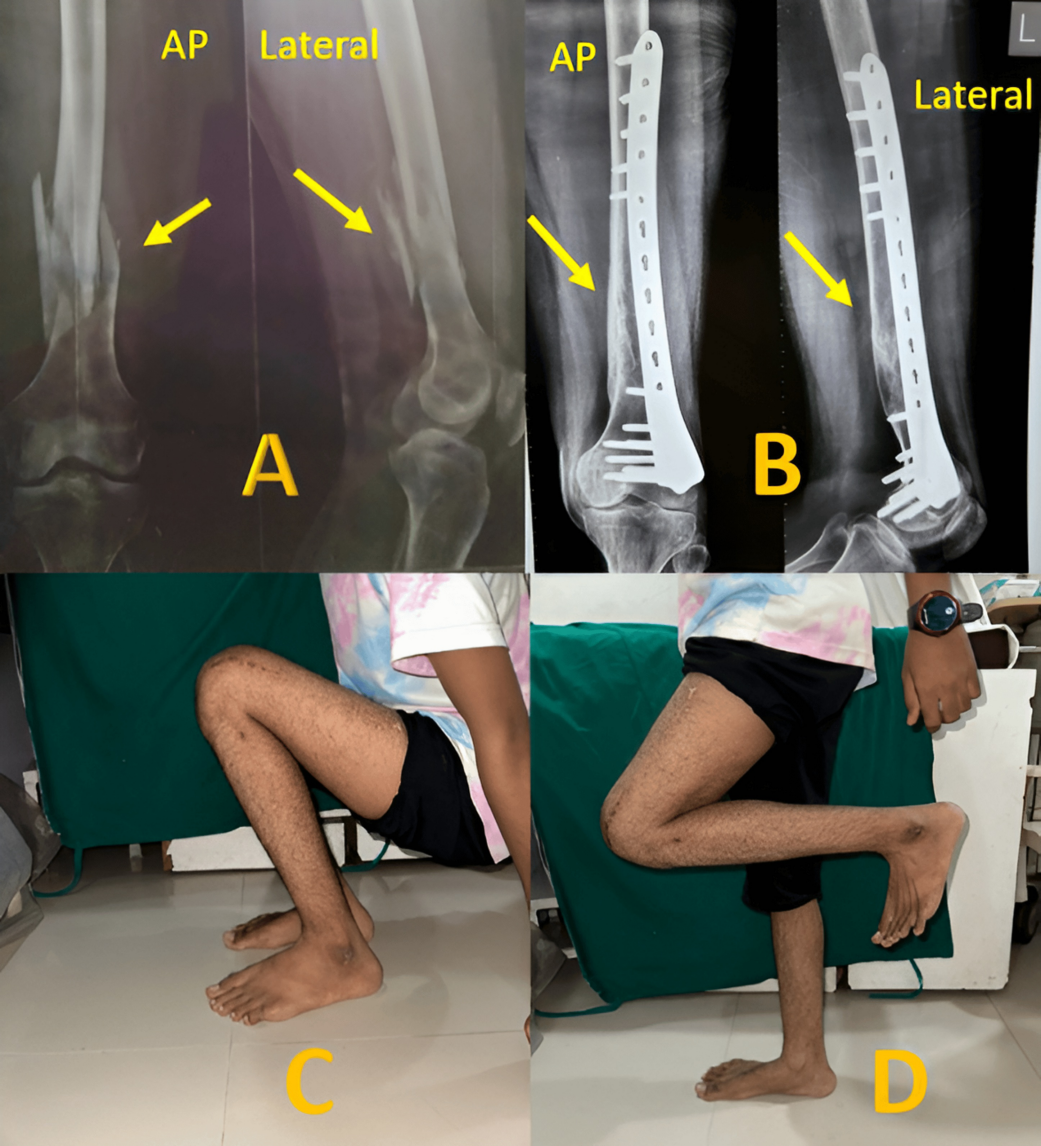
Excellent results obtained with DFLCP (A) AP and lateral X-rays after trauma (arrow showing comminuted fracture), (B) AP and lateral X-rays on the final follow-up (an arrow showing union), (C) Squatting at the final follow-up, and (D) Knee flexion at the final follow-up DFLCP: Distal Femur Locking Compression Plate
